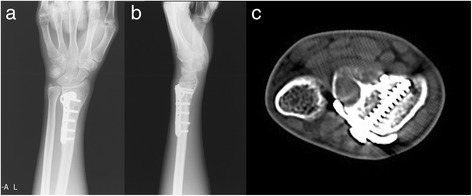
Preoperative anteroposterior and lateral X-ray and CT scans of a 20-year-old man with RGCT of the left distal radius (Campanacci grade III, recurrence at 13 months after curettage) (a-c)
